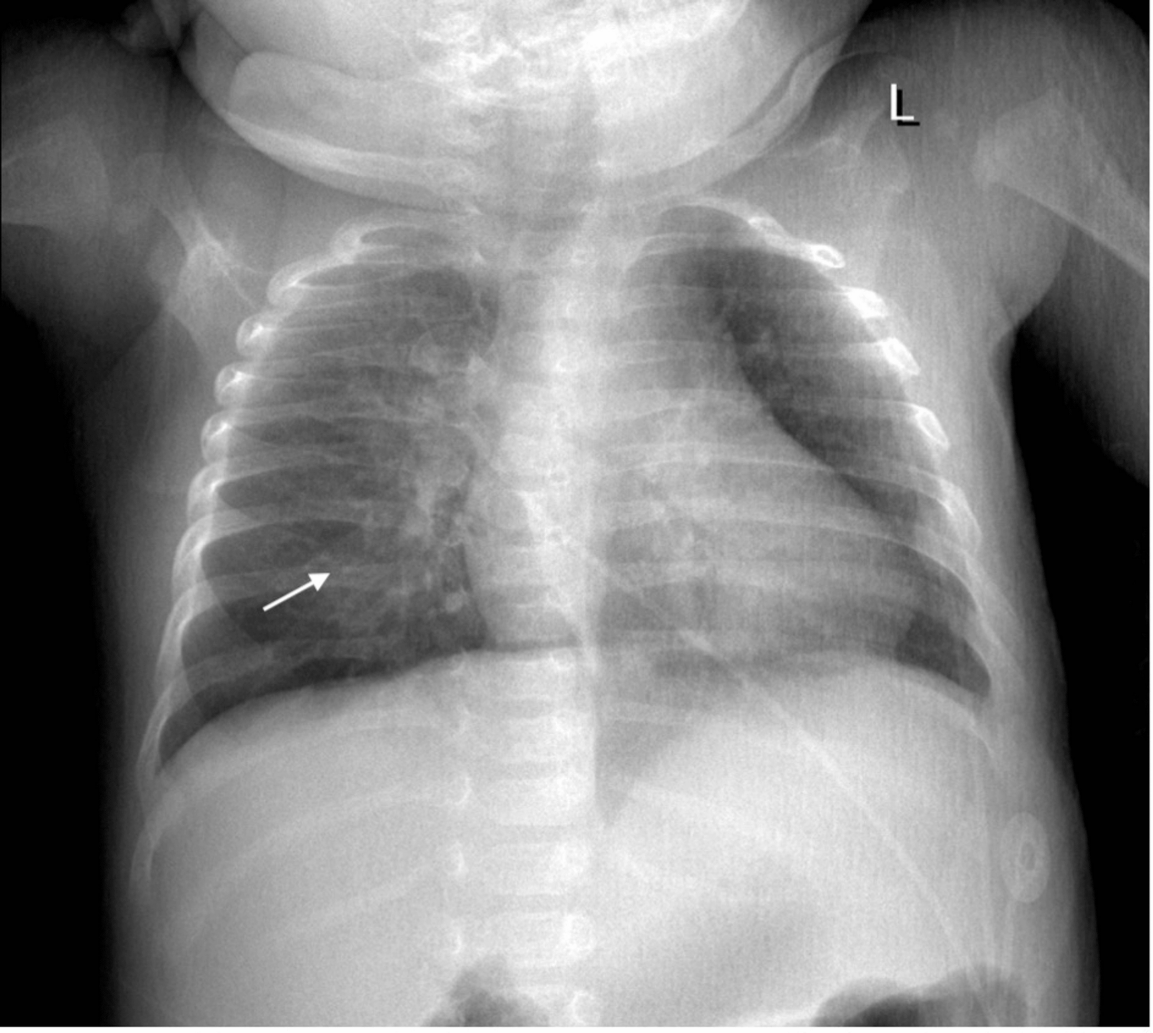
X-ray of the chest showing bilateral patchy airspace opacities as indicated by the white arrow
